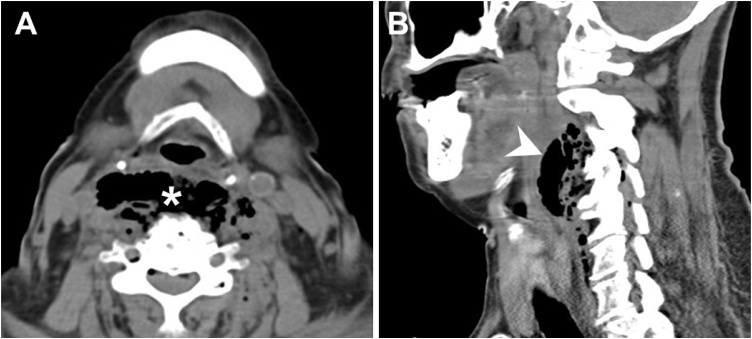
A 65-year-old female patient with deep neck infection. (A) Axial view of the computed tomography image revealing gas collection in the bilateral retropharyngeal, parapharyngeal, and carotid spaces (asterisk). (B) Sagittal view of the computed tomography image presenting diffuse soft-tissue swelling with fluid and air collection in the prevertebral region (arrowhead).
